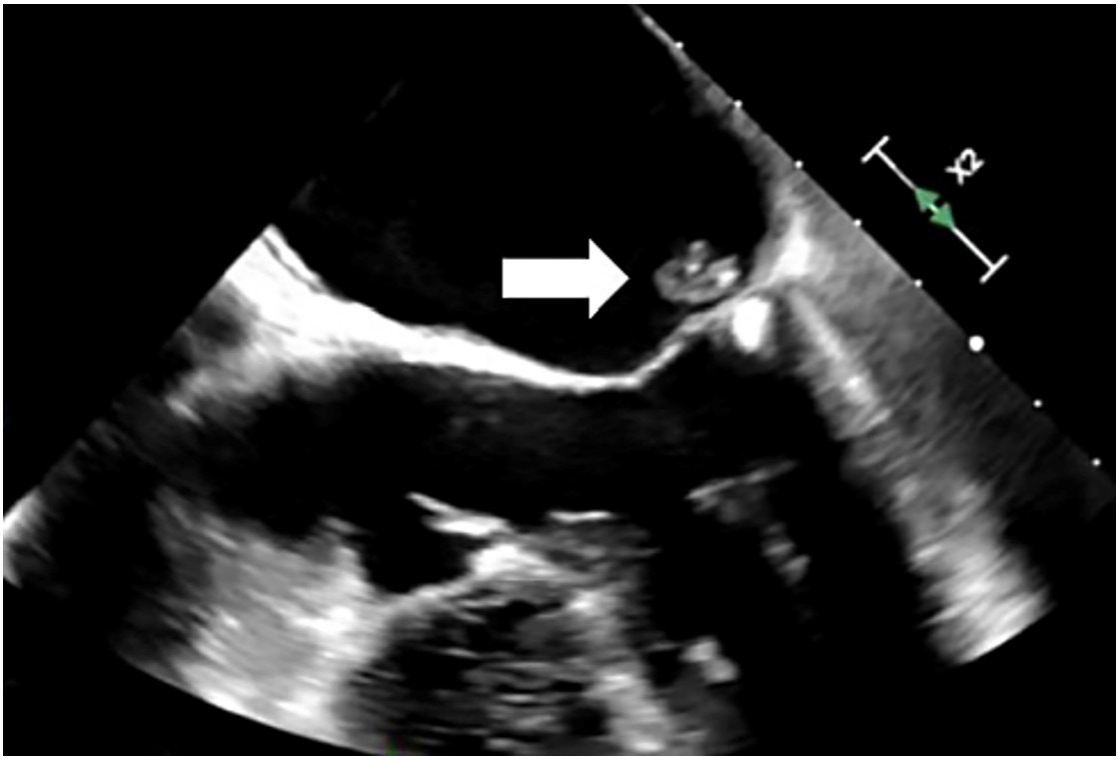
Trans-esophageal echocardiography showing a 12 mm-sized mobile atrial vegetation with marked thickening of the posterior mitral leaflet.
